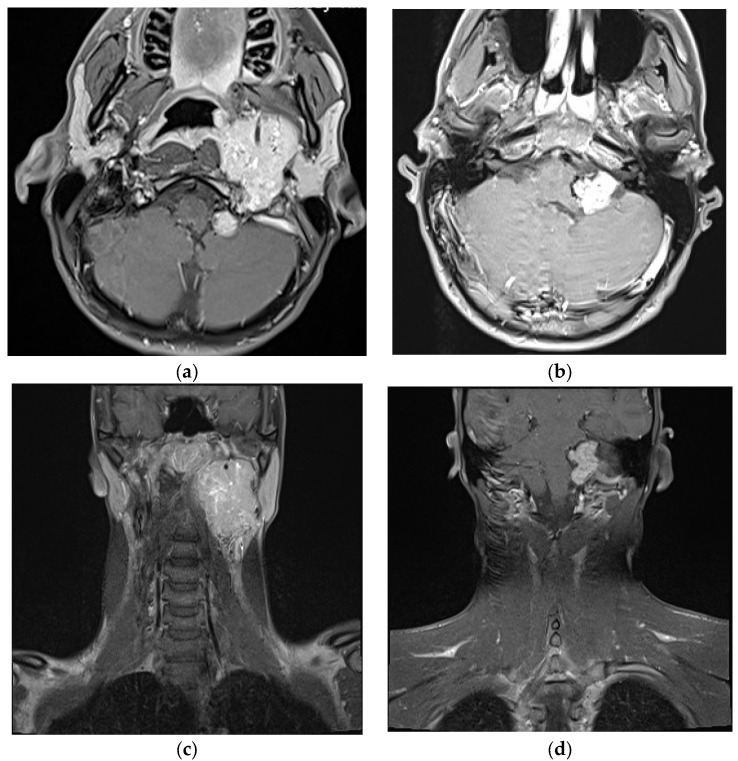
(a–d) T1 contrast enhanced transversal MRI demonstrating the tumor growing from the jugular foramen into the posterior cavity and the upper neck.
